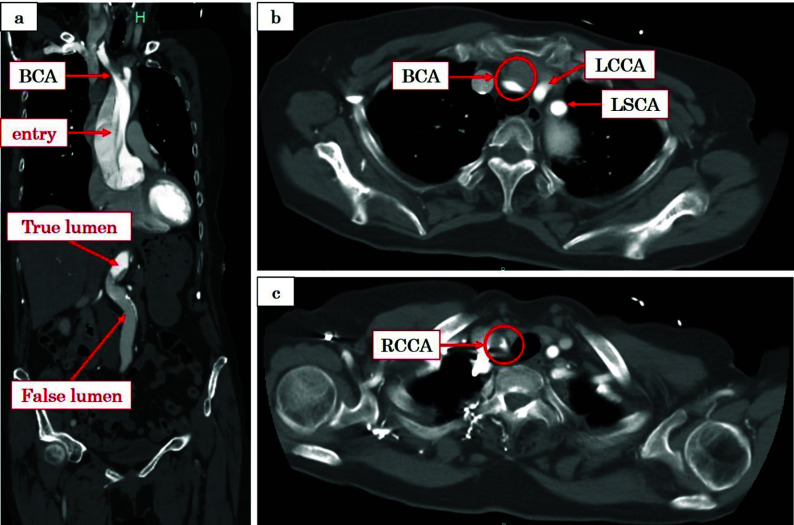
(a) Aortic contrast-enhanced CT scan indicates a DAA with malperfusion of the left lower limb and an entry tear in the ascending aorta. (b) LCCA and LSCA show no abnormalities. (b, c) The true lumen from the BCA to the RCCA is narrowed by the false lumen. CT: Computed tomography; DAA: Dissecting aortic aneurysm; LCCA: Left common carotid artery; LSCA: Left subclavian artery; BCA: Brachiocephalic artery; RCCA: Right common carotid artery.
